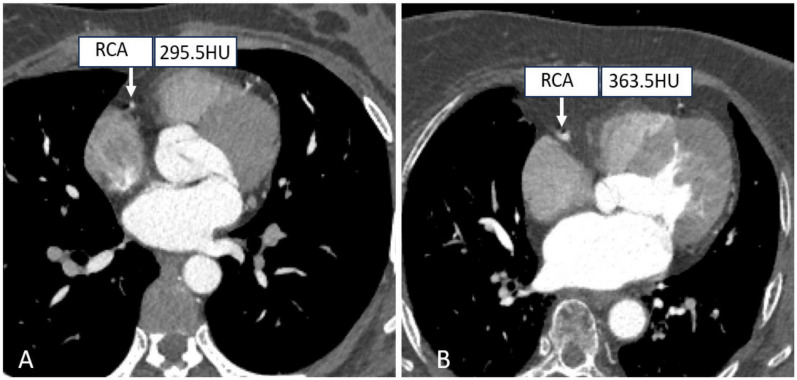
Right coronary CTA images with different tube voltages. ((A) A patient with a tube voltage of 100 kV; (B A patient with a tube voltage of 120 kV.).
